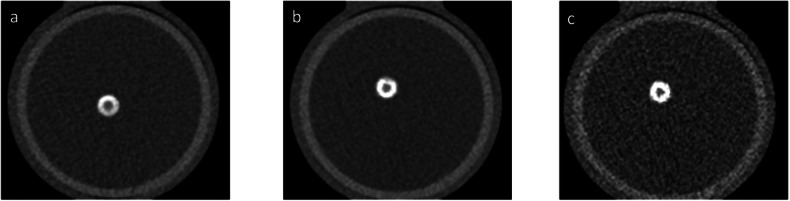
Depiction of non-enhanced CT scan and virtual non-iodine (VNI) reconstructions of the artery: (a) non-enhanced CAC scan – the reference calcium scoring CT scan; (b) VNI CCTA scan reconstructed with FBP, kernel Qr36f, and 3 mm slice thickness; (c) VNI CCTA scan reconstructed with FBP, kernel Qr36f, and 0.4 mm slice thickness. FBP, filtered back projection
